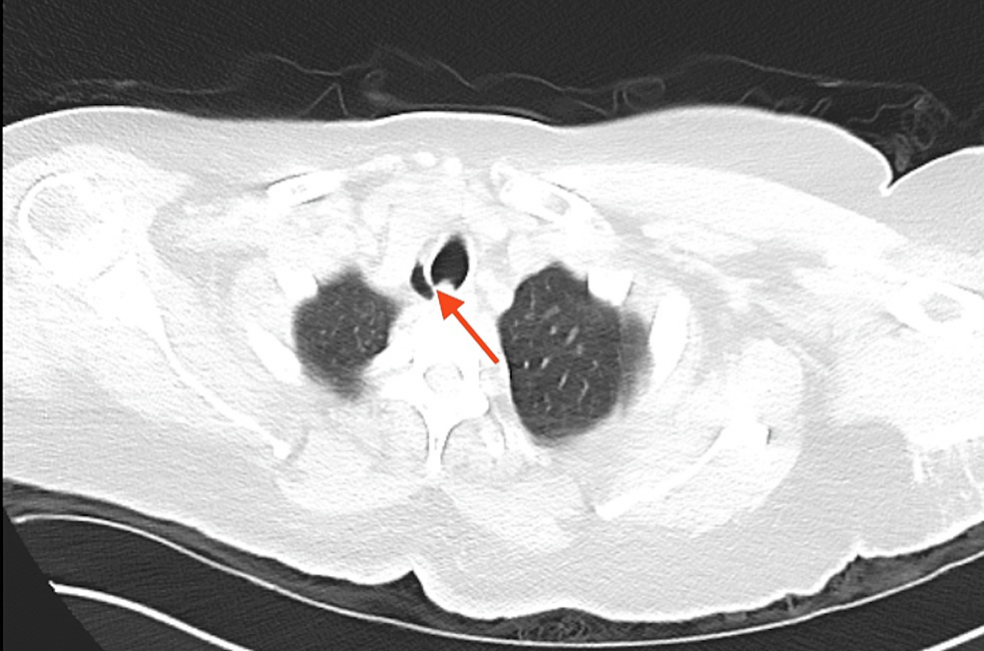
Computed tomography (CT) of the chest without contrast, axial lung window. Red arrow points to tracheal diverticulum, lateral to trachea.
